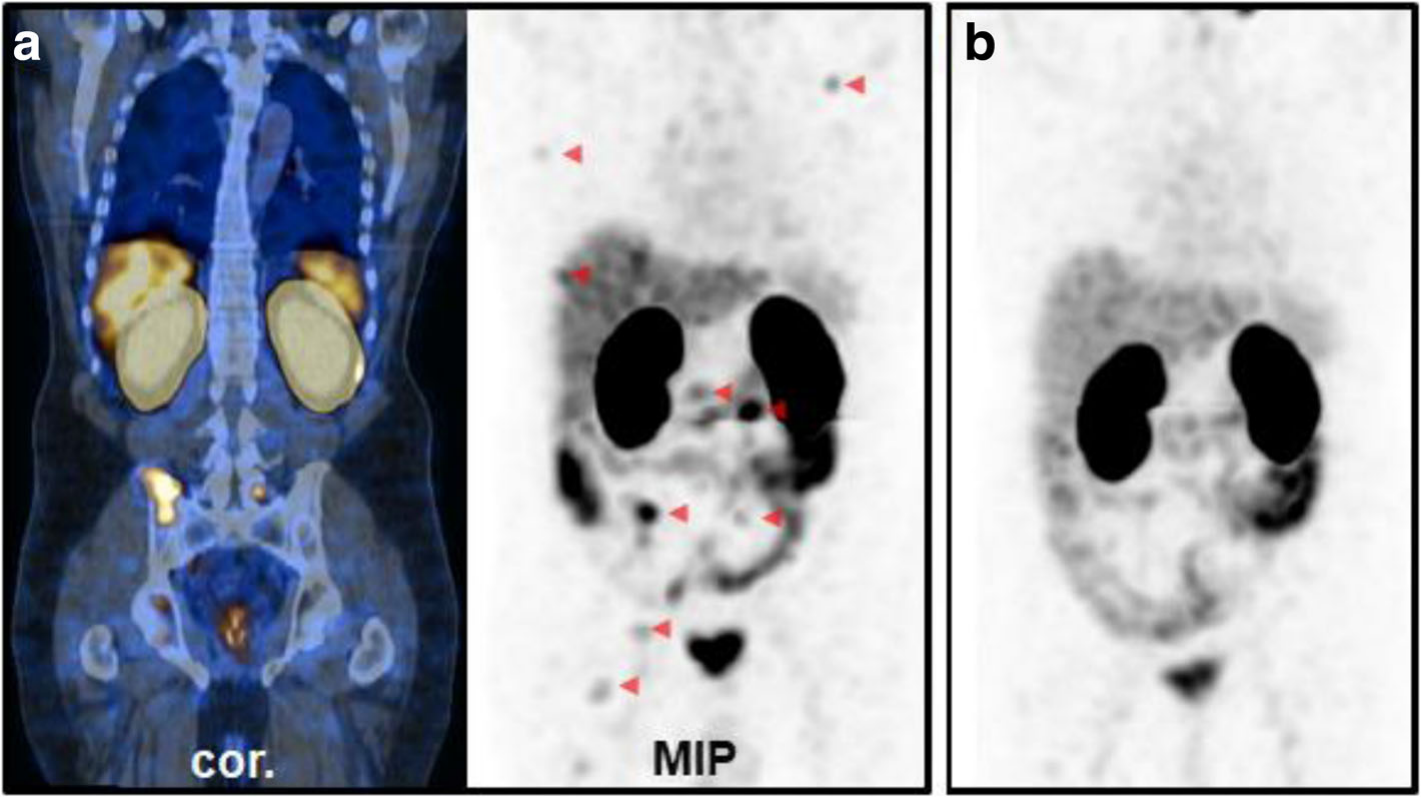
[^99m^Tc]Tc-PSMA-I&S-SPECT/CT of a 59-year-old patient with radical prostatovesiculectomy and salvage radiotherapy. According to morphological imaging 2 years later osseous metastases were suspected. a At baseline, multiple osseous and lymph node metastases (partly marked with red arrows) were detected with mostly mild PSMA expression (PSA 4.75 ng/ml). b Follow-up scan 4 months later under antihormonal therapy (PSA 0.01 ng/ml). [^99m^Tc]Tc-PSMA-I&S-SPECT/CT was negative
